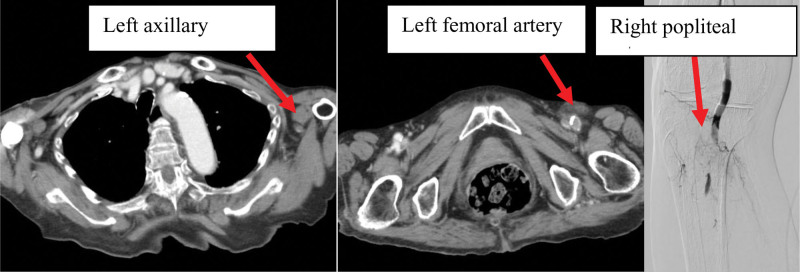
Chest contrast-enhanced computed tomography and lower-extremity angiography at admission. Contrast-enhanced computed tomography and angiography shows obstruction of the left axillary artery, left femoral artery, and right popliteal artery.
